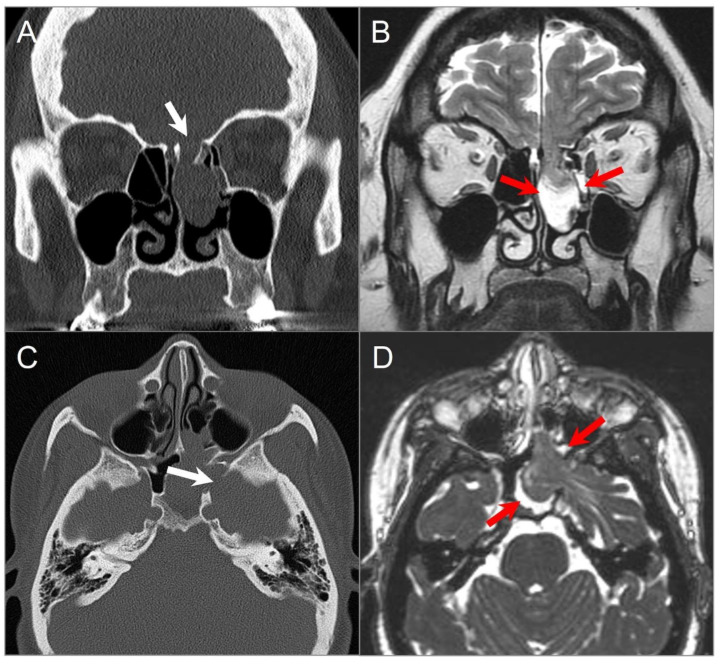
Ethmoidal and sphenoidal menigoencephaloceles. (A) Coronal CT image reveals a bony defect in the left cribriform plate (white arrow), with a well-defined polypoid lesion protruding into the left-sided ethmoid sinus and nasal cavity. (B) Coronal T2W image reveals that the lesion correlates with herniated left frontal lobe parenchyma, CSF, and meninges through the bony defect (red arrows). (C) Axial CT image in a different patient demonstrates a bony defect in the left lateral sphenoid sinus wall (white arrow), with opacification of the left sphenoid sinus and left posterior ethmoid air cells. (D) Axial CISS image shows herniation of the anteromedial left temporal lobe, meninges, and CSF through the bony defect (red arrows).
